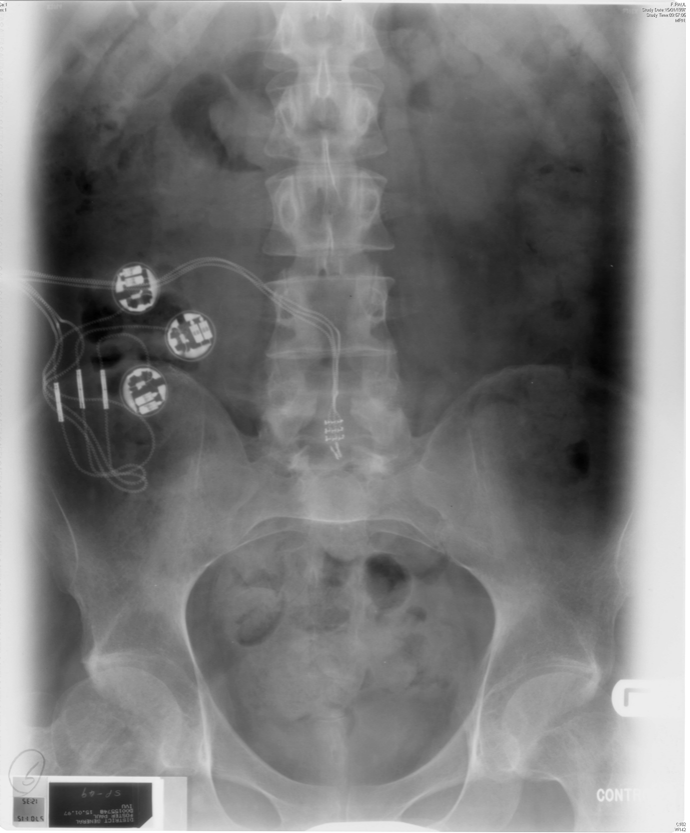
X-ray of abdomen, taken on 15 January 1997, showed all components of Brindley stimulator (receiver block, cables, and electrodes) in place.
